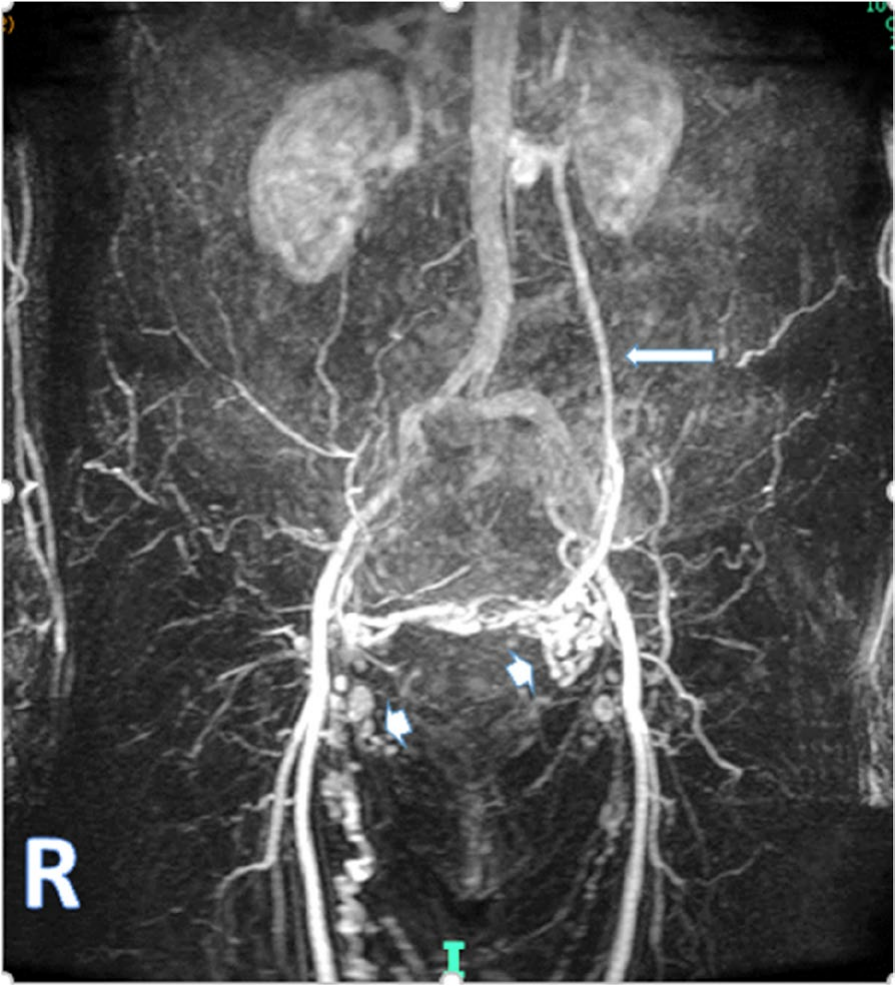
MRI/MRv The left ovarian vein (long arrow) is clearly identified filling early and extends down into the pelvis where small varices (arrow head) are filled on the left side with a crossover of venous flow to the right side and subsequent multiple varicosities are identified in the right upper thigh on this TR IC K S study
